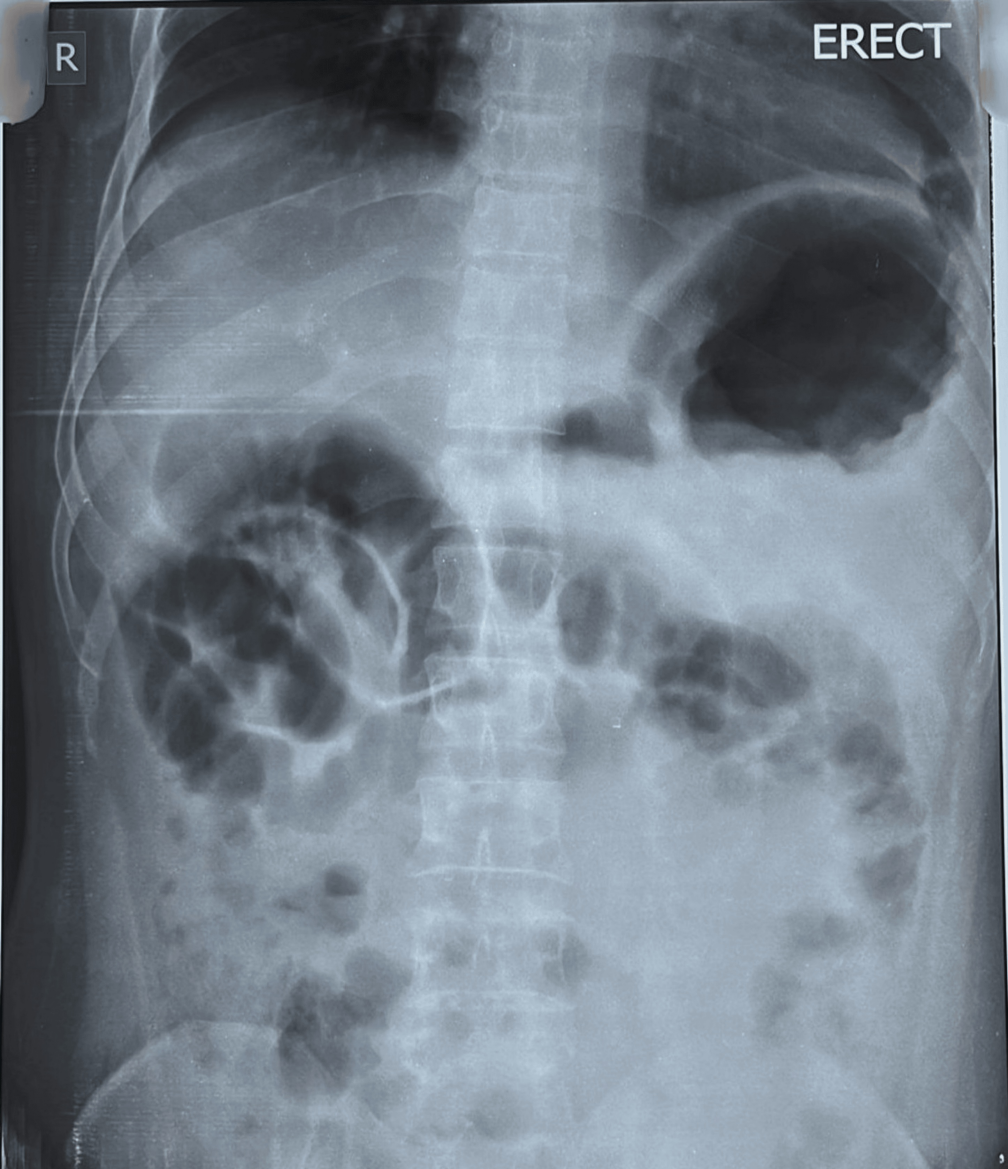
Erect abdominal X-ray showing no signs of obstruction
